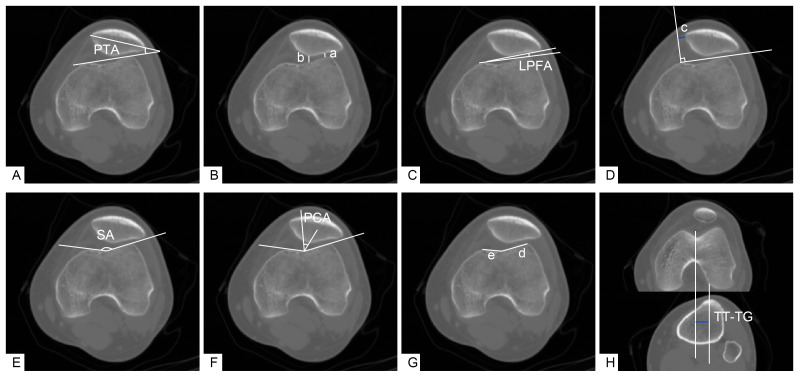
Measurement of assessment indices for patellofemoral instability in knee CT. (A) Patellar tilt angle (PTA): the angle between the line connecting each of the highest points of the medial and lateral femoral condyles and the transverse axis of the patella. (B) Patellofemoral index (PFI): the ratio of the narrowest width of the medial patellofemoral joint space (b) to the narrowest width of the lateral patellofemoral joint space (a). (C) Lateral patellofemoral angle (LPFA): the angle between the tangent line of the lateral articular surface of the patella and a line connecting each of the highest points of the medial and lateral femoral condyles. (D) Lateral patellar displacement (LPD): the length between a vertical line connecting each of the highest points of the medial and lateral femoral condyles from the highest point of the medial femoral condyle (c) and the inner edge of the patella. (E) Sulcus angle (SA): the angle between the line connecting the lowest point of the femoral trochlear groove and each of the highest points of the medial and lateral trochlear articular surfaces. (F) Patellar congruency angle (PCA): the angle between a line connecting the lowest point of the femoral trochlear sulcus and the lowest point of the patellar crest and the bisector of the SA made on the axial image of the patella [14]. (G) Sulcus lateral facet ratio (SLFR): the ratio of the lateral articular surface of the femoral trochlear joint (d) to the medial articular surface of the femoral trochlear joint (e). (H) Tibial tubercle-trochlear groove distance (TT-TG): the mediolateral distance between the highest point of the tibial tuberosity to the deepest point of the trochlear groove in line with the posterior condylar axis.
